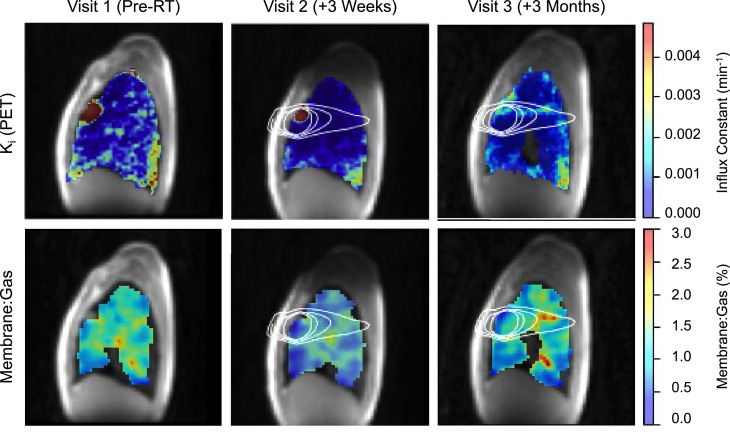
Net influx constant (K_i_) and membrane:gas spatial maps. Spatial maps describing ^18^F-FDG (top) and HP-Xe (bottom) uptake in the lung tissues overlayed on ^1^H MRI before, and 3 weeks and 3 months following, completion of RT. The 10-, 20-, 30-, and 40-Gy isodose curves are shown. Decreased ^18^F-FDG and Membrane:Gas is observed across the lung 3 weeks after therapy (middle column). Increased ^18^F-FDG and Membrane:Gas is observed in the beam path between 10 and 20 Gy isodose curves 3 months after RT (right column). *Abbreviations:*^18^F-FDG = fluorodeoxyglucose; PET = positron emission tomography; RT = radiation therapy.
